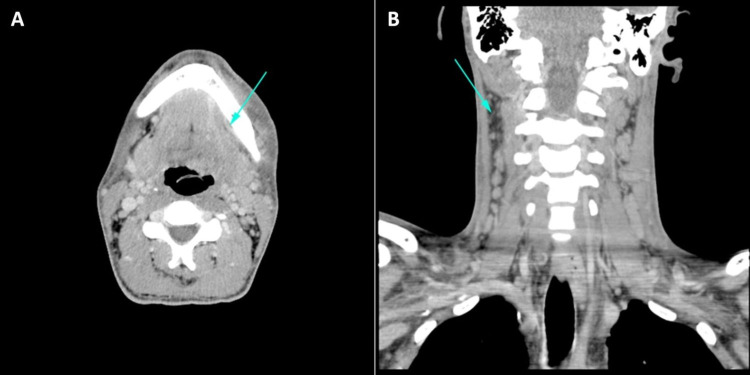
Computed tomography of the head and neck on admission with intravenous contrast. Axial section (A) showing a small contrast-enhancing mass consistent with abscess (arrow). Coronal section (B) showing extensive reticulation within the subcutaneous soft tissues, reflecting diffuse cellulitis (arrow).
